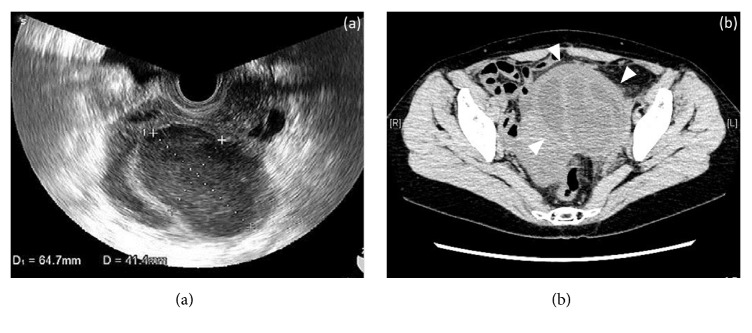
(a) Transvaginal ultrasonography at the first visit of the patient, showing a cystic tumor in the left adnexal area. (b) Pelvic CT on day 8 of hospitalization, showing that the infectious left ovarian cyst had grown to 10 cm (arrowheads).
